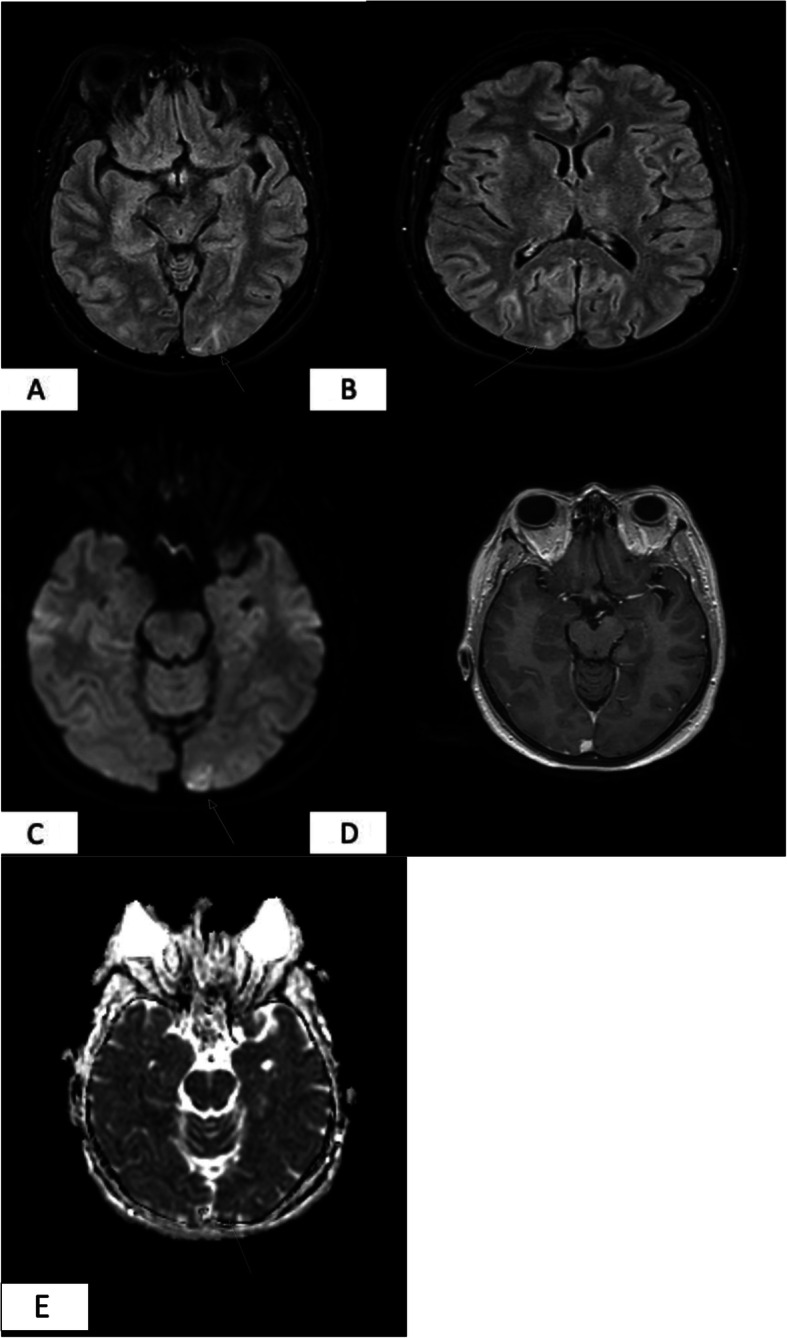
a+b: Axial FLAIR image showing cortical hyperintensity in both occipital lobes. a- Left; b- Right; c. DWI image showing restricted diffusion in left occipital cortex; d Axial T1 post Gadolinium image showing no pathological enhancement. e- ADC map showing mild diffusion restriction
